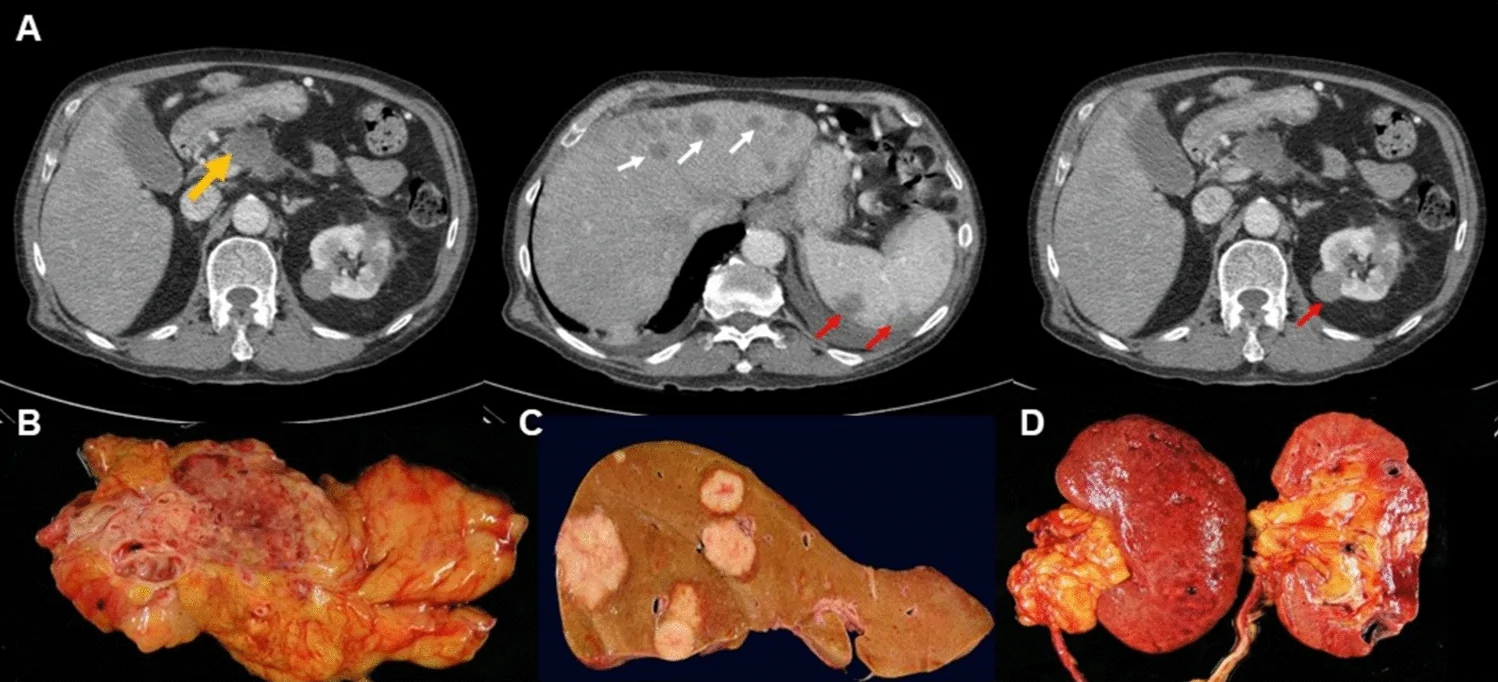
A Abdominal CT scan showing a pancreatic lesion compatible with a tumor (orange arrow), together with liver metastases (white arrows) and splenic and renal infarcts (red arrows). B-D Macroscopic autopsy photographs of the same patient showing the pancreatic tumor (A), the liver with metastases (B) and the kidney with areas of infarction
